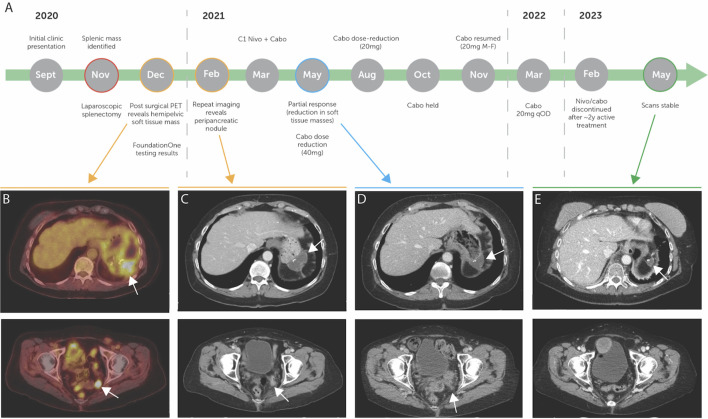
(A) Diagnosis and treatment timeline of the patient, with pre-treatment imaging highlighted in yellow, on treatment imaging highlighted in blue, and post-treatment imaging highlighted in green. (B) Pre-treatment PET scan showing FDG avid peripancreatic fluid collection and FGD avid pelvic soft tissue mass. (C) Pre-treatment CT scan showing peripancreatic nodule and redemonstrating pelvic soft tissue mass. (D) On-treatment CT scan showing partial response in peripancreatic and pelvic soft tissue masses. (E) Post-treatment CT scan demonstrating stable disease. (B–E) white arrows demonstrate highlighted areas of interest.
